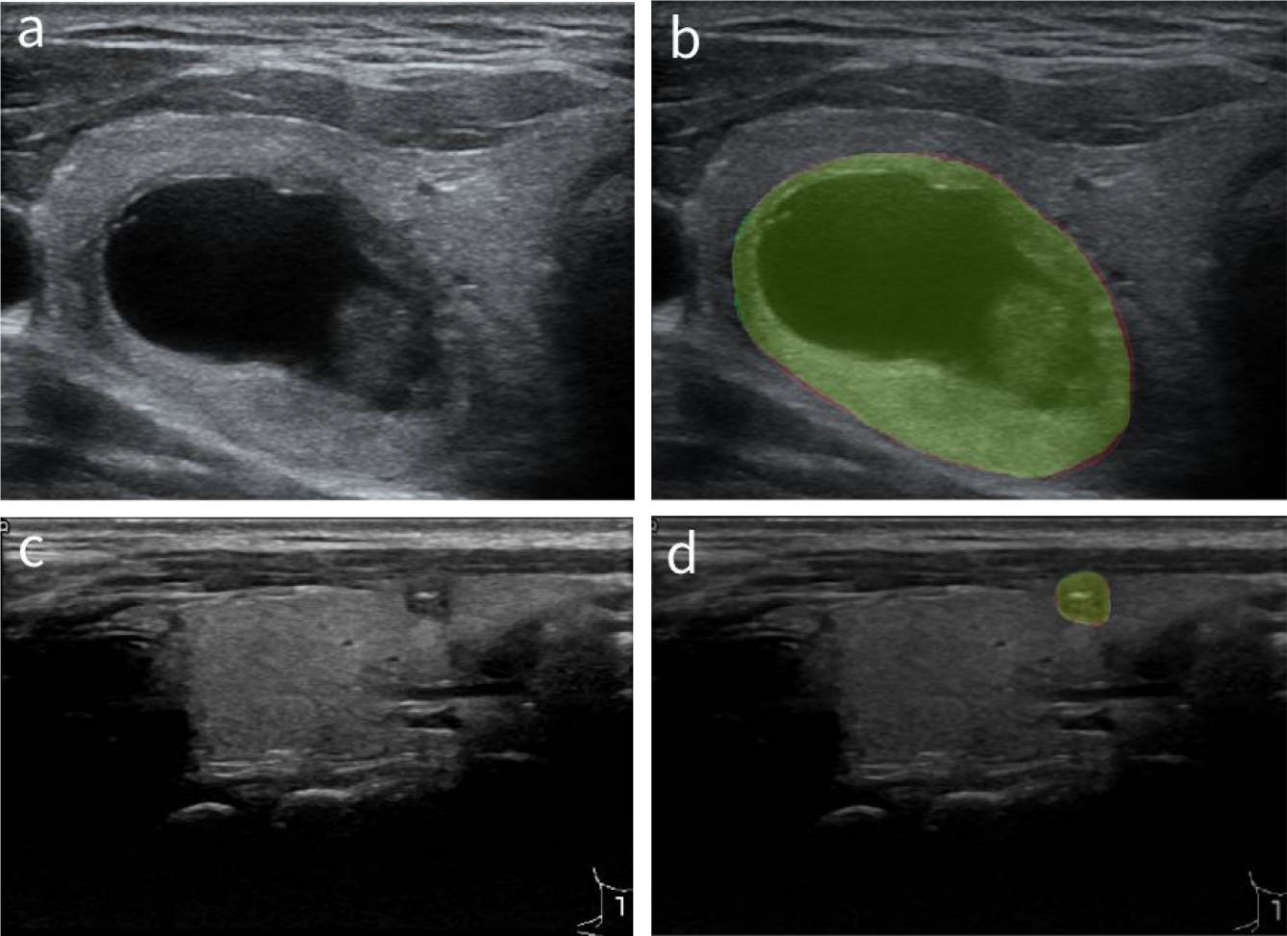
Comparison of the masks generated by the AI system and the masks outlined by the radiologists. (A, C) The original thyroid ultrasound images. (B, D) Masks generated by the AI system and radiologists, where the red segments are produced by the AI system, and the green ones are manually drawn by the radiologists. AI, artificial intelligence.
